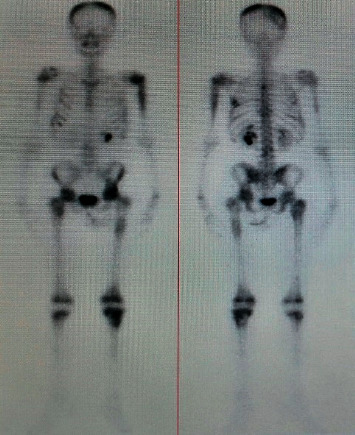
Skeletal scintigraphy showing multiple bone metastases.
